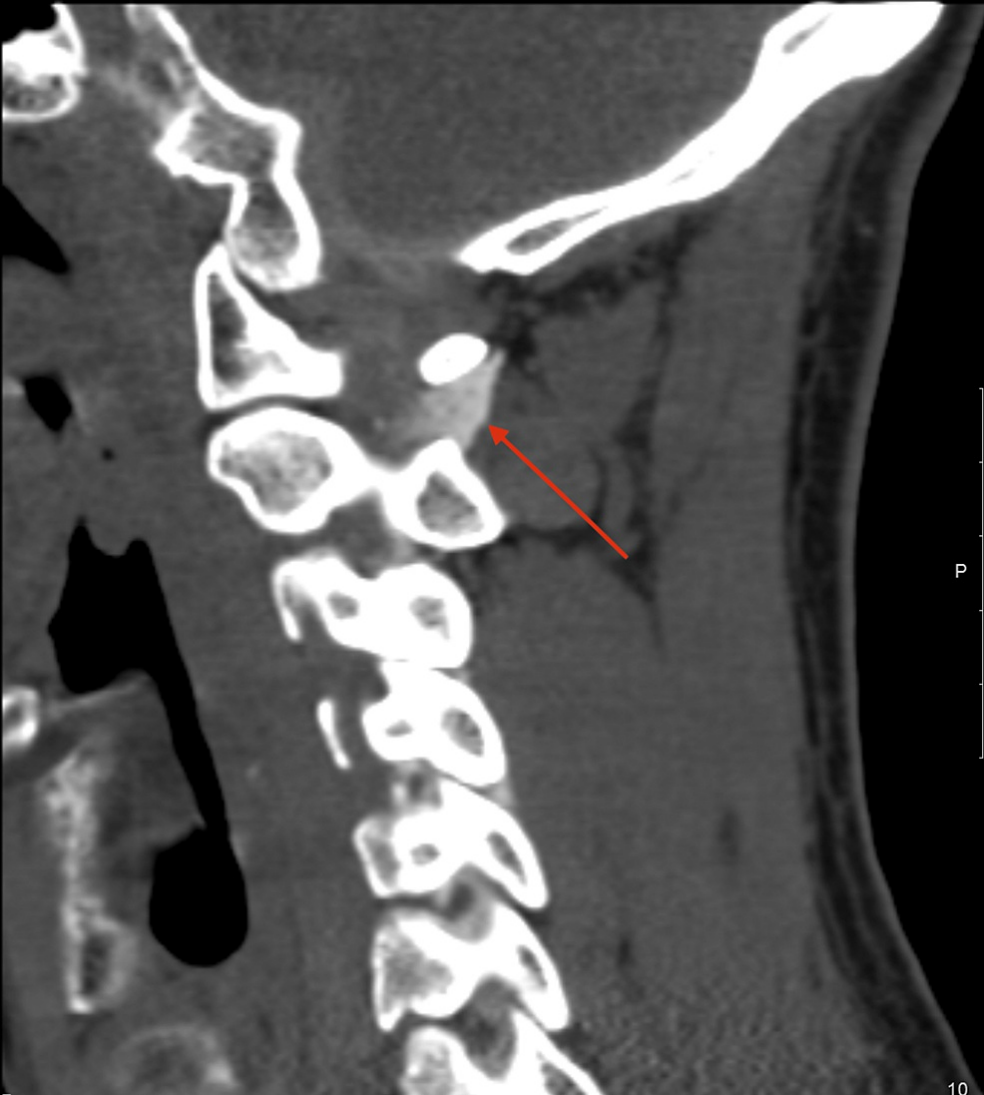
Parasagittal cervical myelo-CT showing contrast outside the subarachnoid space (red arrow), as evidence of a right-sided C1-C2 CSF fistula.
